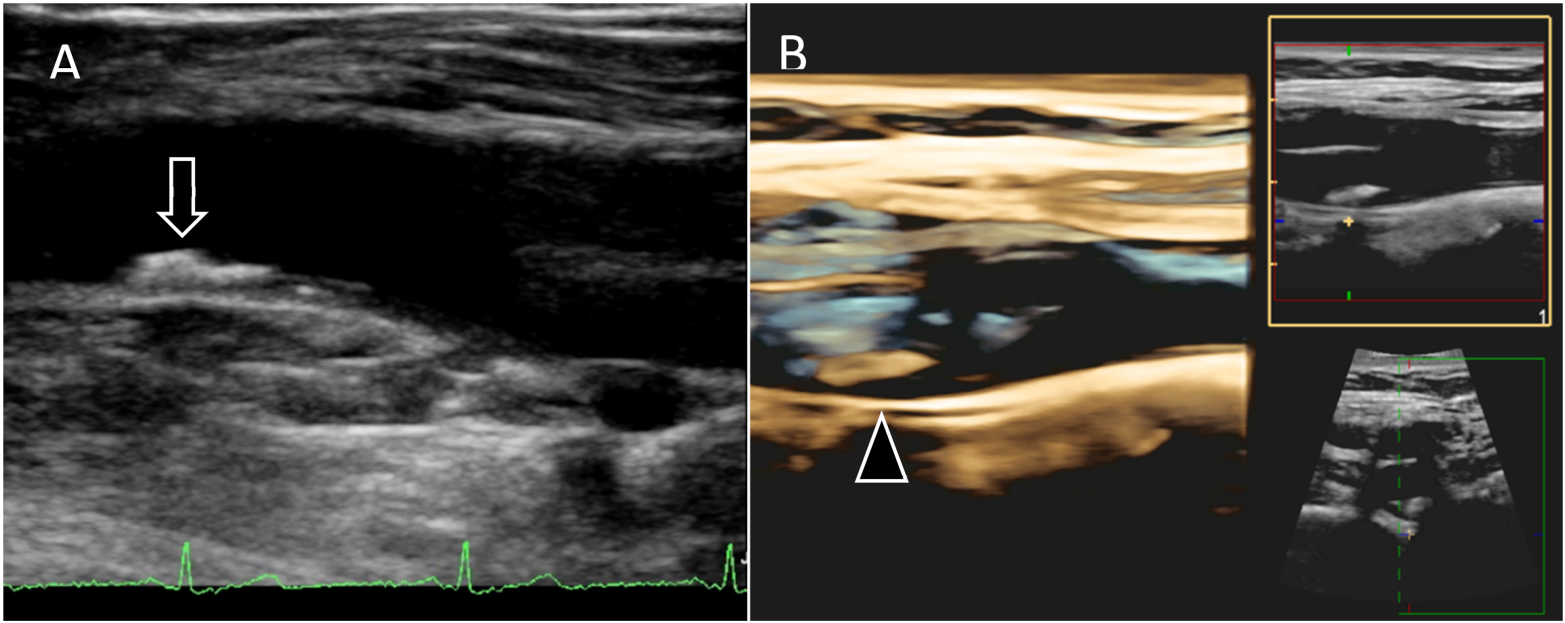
2D and 3D vascular ultrasound (A) 2D images of femoral artery showing atherosclerotic plaque (arrow) located in the common femoral artery prior to bifurcation. (B) 3D images of carotid artery bifurcation showing atherosclerotic plaque (arrow head) located in internal carotid artery.
